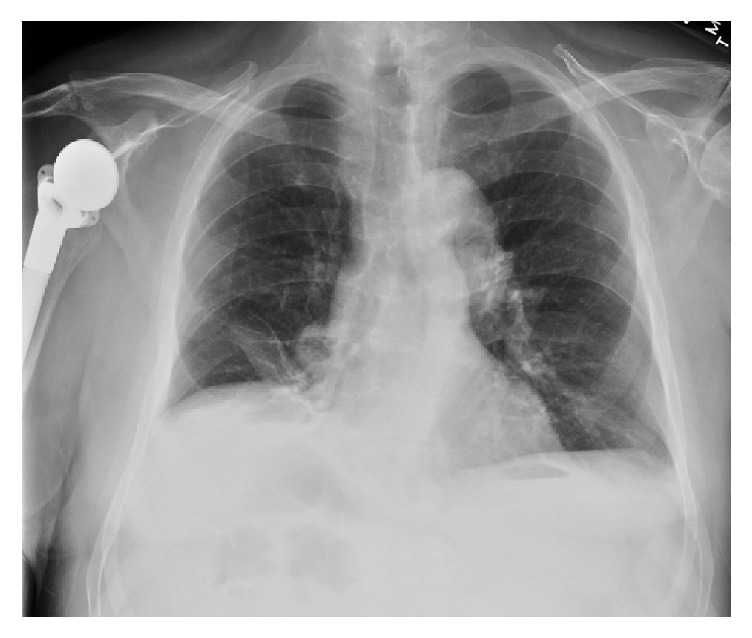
Chest X-ray 6 weeks postoperatively.
